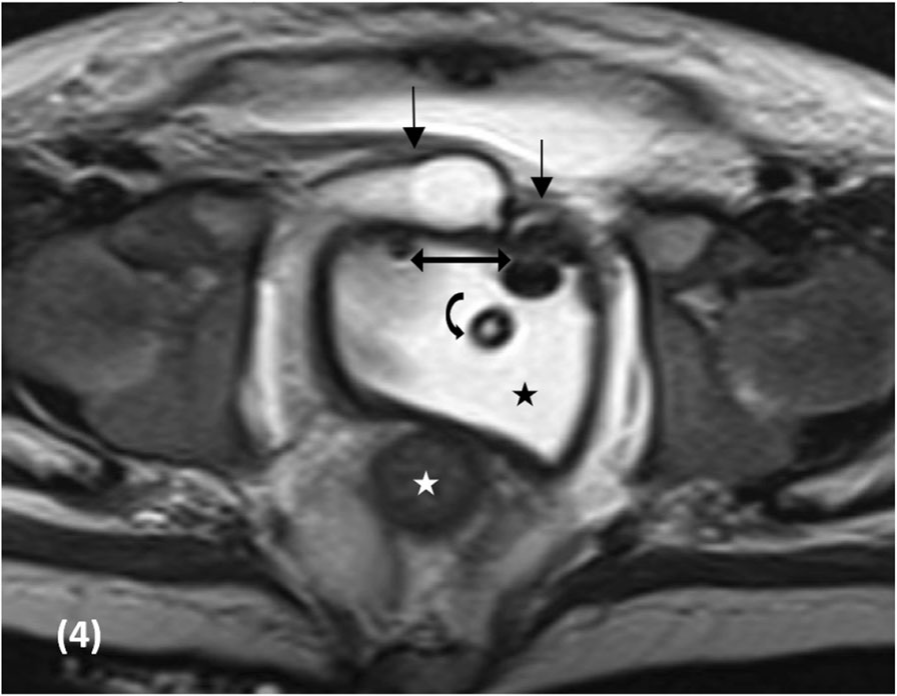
Axial T2 MRI of the pelvis at the level of the rectum (star), showing abnormal anteriorly directed bilateral distal ureters (black arrows), lying superficially posterior to the anterior abdominal wall, inserting into the vagina, close to the midline. The vagina is distended with fluid (black star) and contains a Foley catheter (curved black arrow). There are two foci of air in the vagina (double sided arrow)
